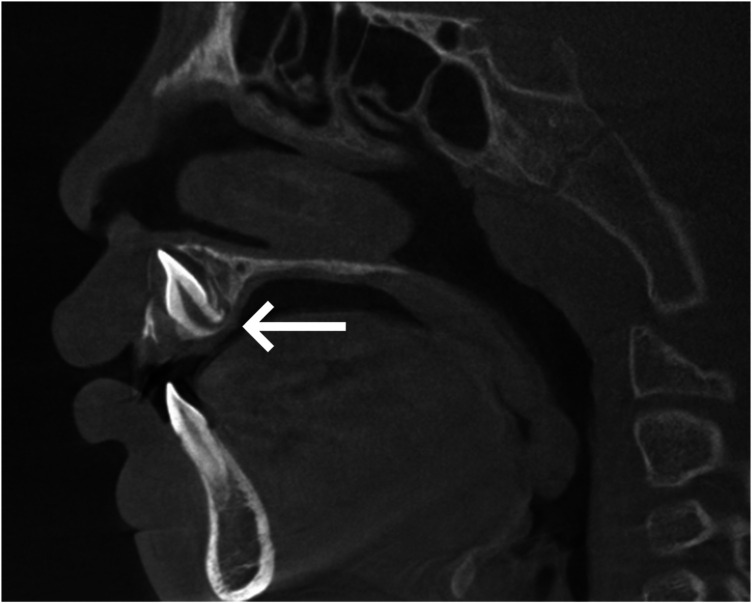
Pre-treatment CBCT. Tooth 11 was dilacerated and severely labially impacted, with a crown-root angulation of approximately 90 degrees (white arrow).
